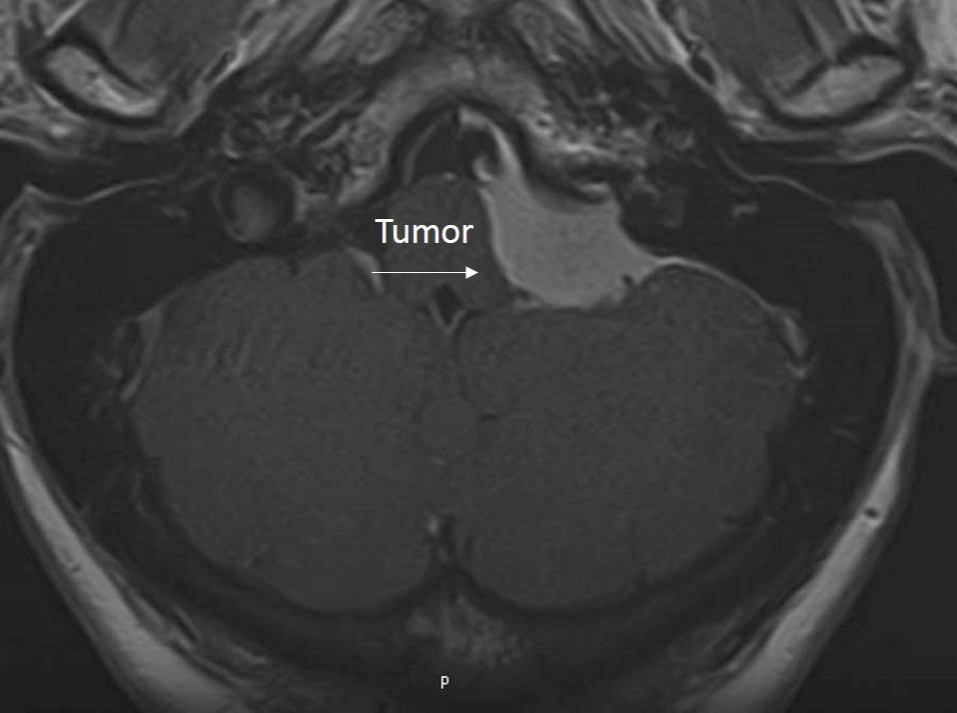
Preoperative MRI of Case 2 Preoperative MRI with contrast depicting a homogenously enhancing lesion with a characteristic dural tail. The lesion is located near the jugular foramen with a component within the cerebellopontine angle.
